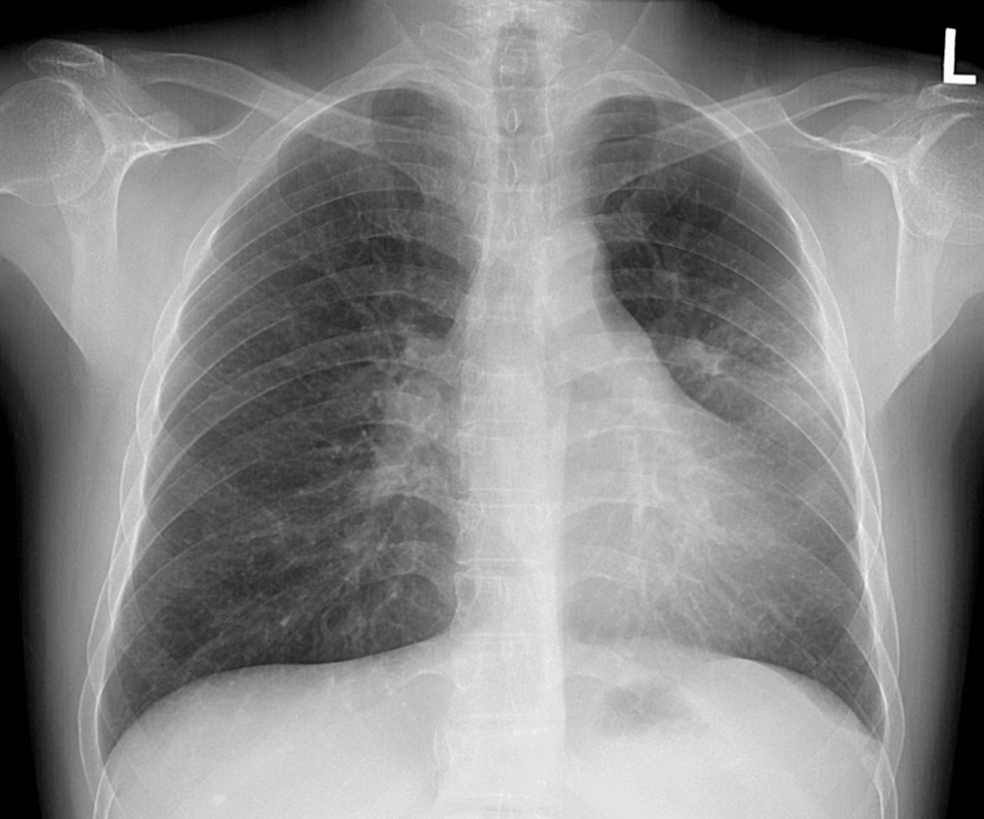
AP chest X-ray depicting LUL opacity (October 18, 2011) AP: anteroposterior; LUL: left upper lobe
